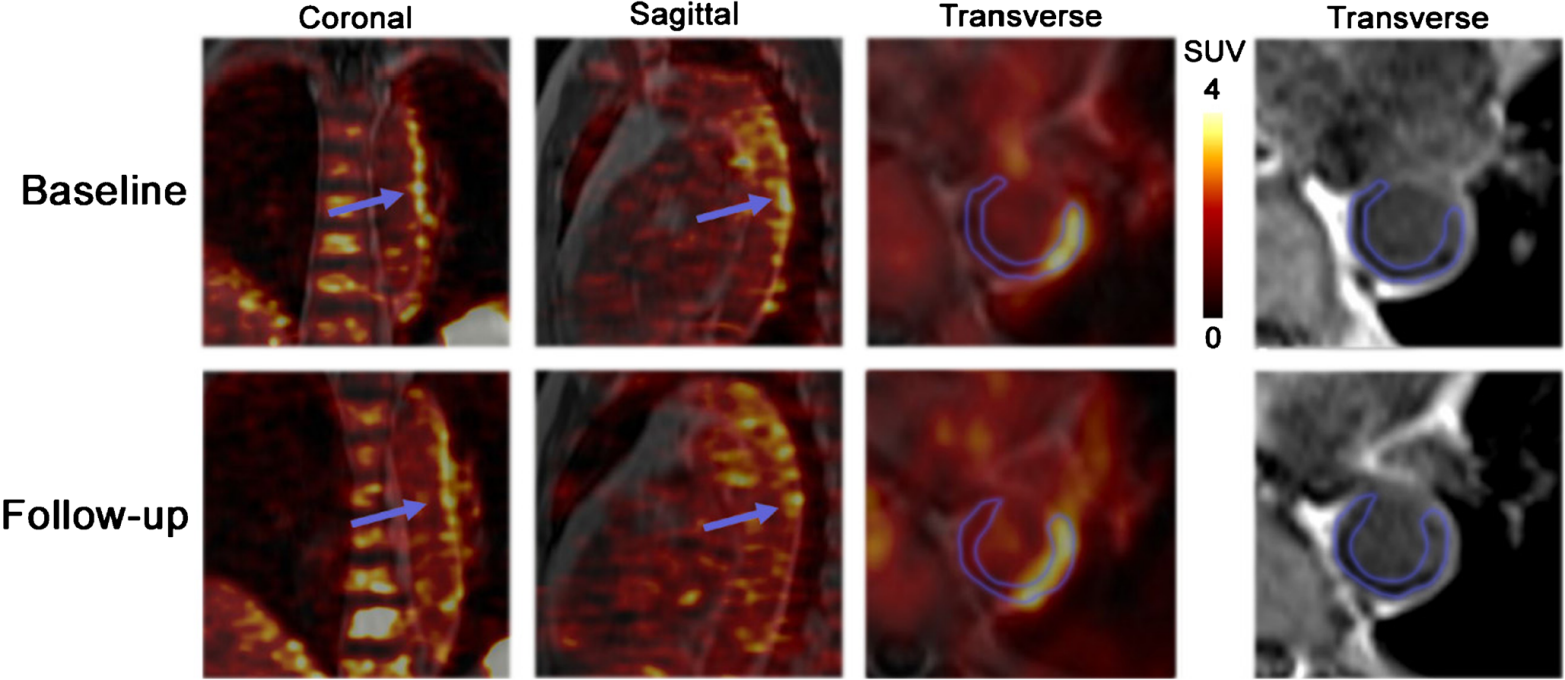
Reproducibility of [^68^Ga]Pentixafor PET imaging in atherosclerotic plaques from a representative scan of a 76-year-old female lymphoma patient. Co-localized uptake in both baseline and follow-up scans was observed (time interval = four months). Transverse view of PET/MRI scan showed spatial accumulation of [^68^Ga]Pentixafor within the mixed atherosclerotic lesions in the descending aortas
